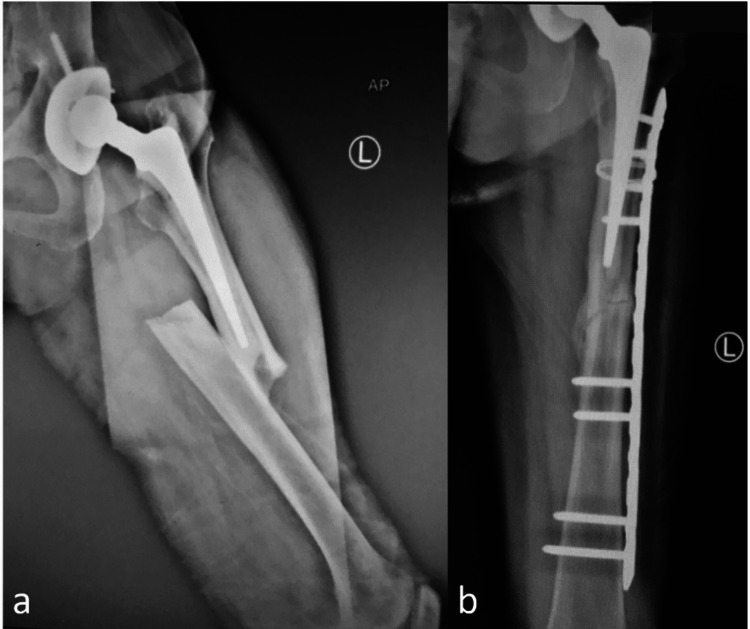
The radiographs from Case 5 a) complete transverse fracture with medial spike with clinical thigh pain at presentation; b) post-operative ORIF with plate and screws with cable wiring four months after fixation.
